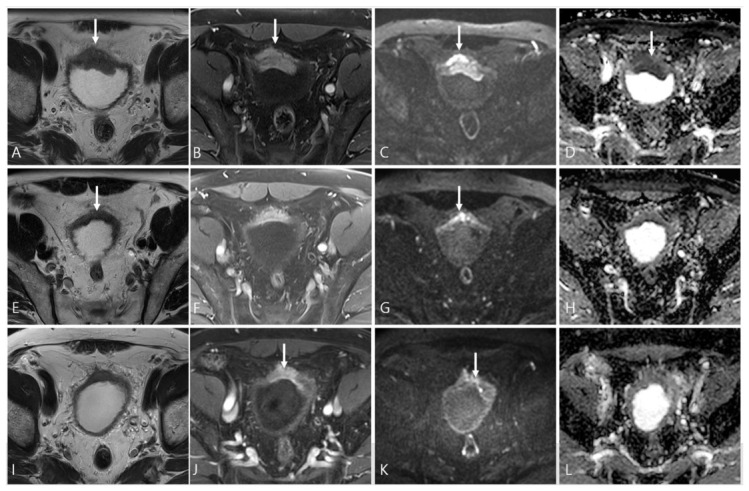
VI-RADS 5—Muscle-invasive bladder cancer with perivesical extension: treatment response monitoring with mpMRI. An 82-year-old male presenting with a bulky infiltrating mass at the anterior dome of the bladder. Sequential mpMRI was performed at baseline, during concurrent chemoradiotherapy (CCRT), and prior to radical cystectomy to monitor treatment response. Baseline MRI (A–D): (A) Axial T2-weighted imaging (T2WI) demonstrates bulky wall thickening with perivesical infiltration at the anterior dome, measuring up to 4.3 cm, with the disruption of the muscularis propria and ill-defined perivesical fat planes. (B) T1-weighted contrast-enhanced imaging (T1CE) shows the relatively homogeneous early enhancement of the lesion extending into the perivesical fat, consistent with extravesical tumor extension. (C) High b-value diffusion-weighted imaging (DWI; b = 1000 s/mm^2^) demonstrates marked diffusion restriction throughout the mass. (D) Apparent diffusion coefficient (ADC) map confirms significantly reduced ADC values. The overall baseline imaging features are consistent with a VI-RADS score of 5, indicating definitive muscle invasion with perivesical tumor extension (≥T3). Mid follow-up MRI after CCRT (E–H): (E) T2WI shows a reduction in the overall extent of the lesion following CCRT, with partial decrease in wall thickening. (F) T1CE demonstrates persistent significant enhancement within the residual lesion, raising strong concern for viable residual tumor. (G) DWI (b = 1000 s/mm^2^) reveals persistent diffusion restriction within the residual mass (arrow). (H) ADC map shows persistently reduced ADC values, further supporting the presence of residual viable tumor despite partial morphological response. Pre-operative MRI (I–L): (I) T2WI demonstrates further subtle reduction in lesion size; however, residual abnormal wall thickening with perivesical changes persists. (J) T1CE shows continued significant enhancement of the residual lesion (arrow). (K,L) DWI and ADC map reveal persistent moderate diffusion restriction, with a reduced extent compared to the prior study. Despite a partial morphological response on sequential imaging, the persistent significant enhancement and diffusion restriction were strongly suggestive of residual viable tumor. Based on the nacVI-RADS scoring system [43], these findings correspond to category 4 (partial response with persistent muscle-invasive disease). Subsequent radical cystectomy confirmed pathological stage ypT3b urothelial carcinoma, validating the mpMRI-based assessment of incomplete response to CCRT.
